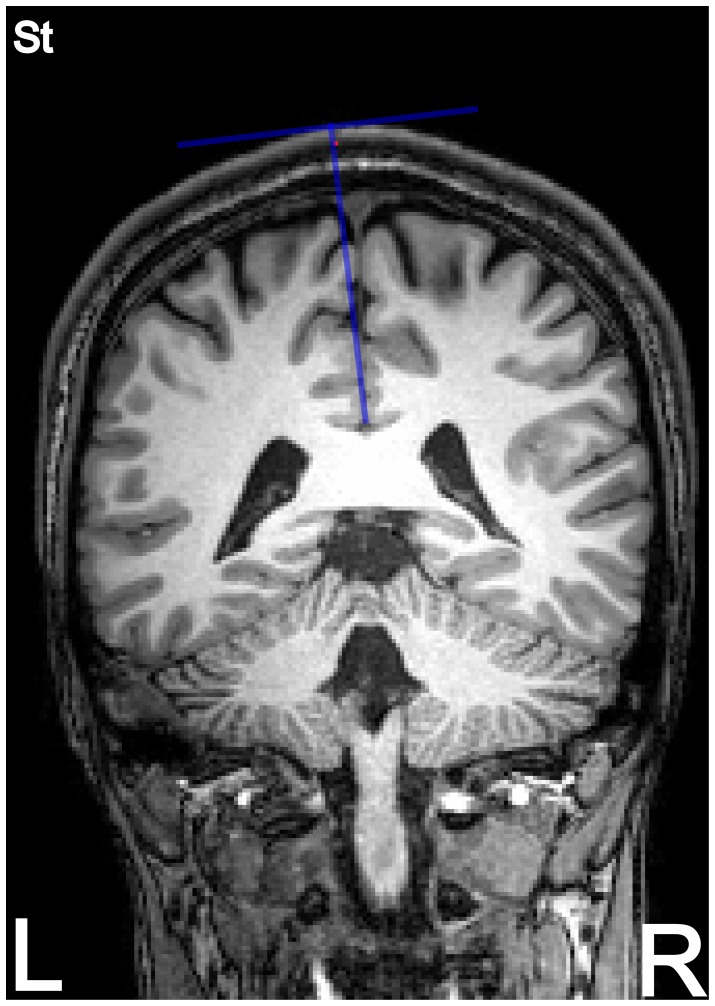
Coronal view of a structural MRI at hot-spot level. From the hot-spot (red dot) at the skull the normal to the skalp surface is extended into the brain. The intersection of this line with the precentral gyrus is used for later evaluation of the angle of the precentral gyrus with respect to the interhemispheric cleft (cf. Figure 8).
